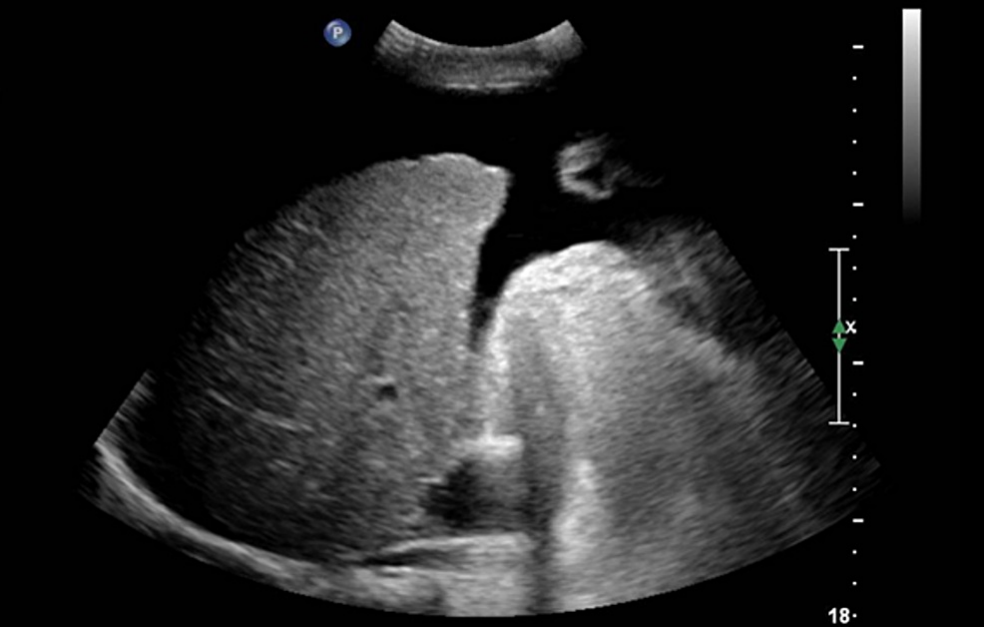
Ultrasound of right upper abdominal quadrant before transjugular intrahepatic portosystemic shunt demonstrating mild ascites.
